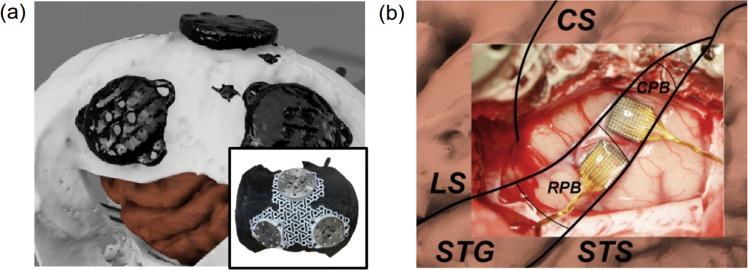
The anatomical structure of the cortex guided posistions of MEA on parabelt. a A 3D model of the skull, brain, and anchoring metal footplates (constructed by merging MRI and CT imaging). Also, a titanium mesh on a 3D-printed skull model. b Photo of the exposed area of the auditory cortex with labels added for relevant cortical structures (CS, central sulcus; LS, lateral sulcus; STG, superior temporal gyrus; STS, superior temporal sulcus; RPB, rostral parabelt; CPB, caudal parabelt).
